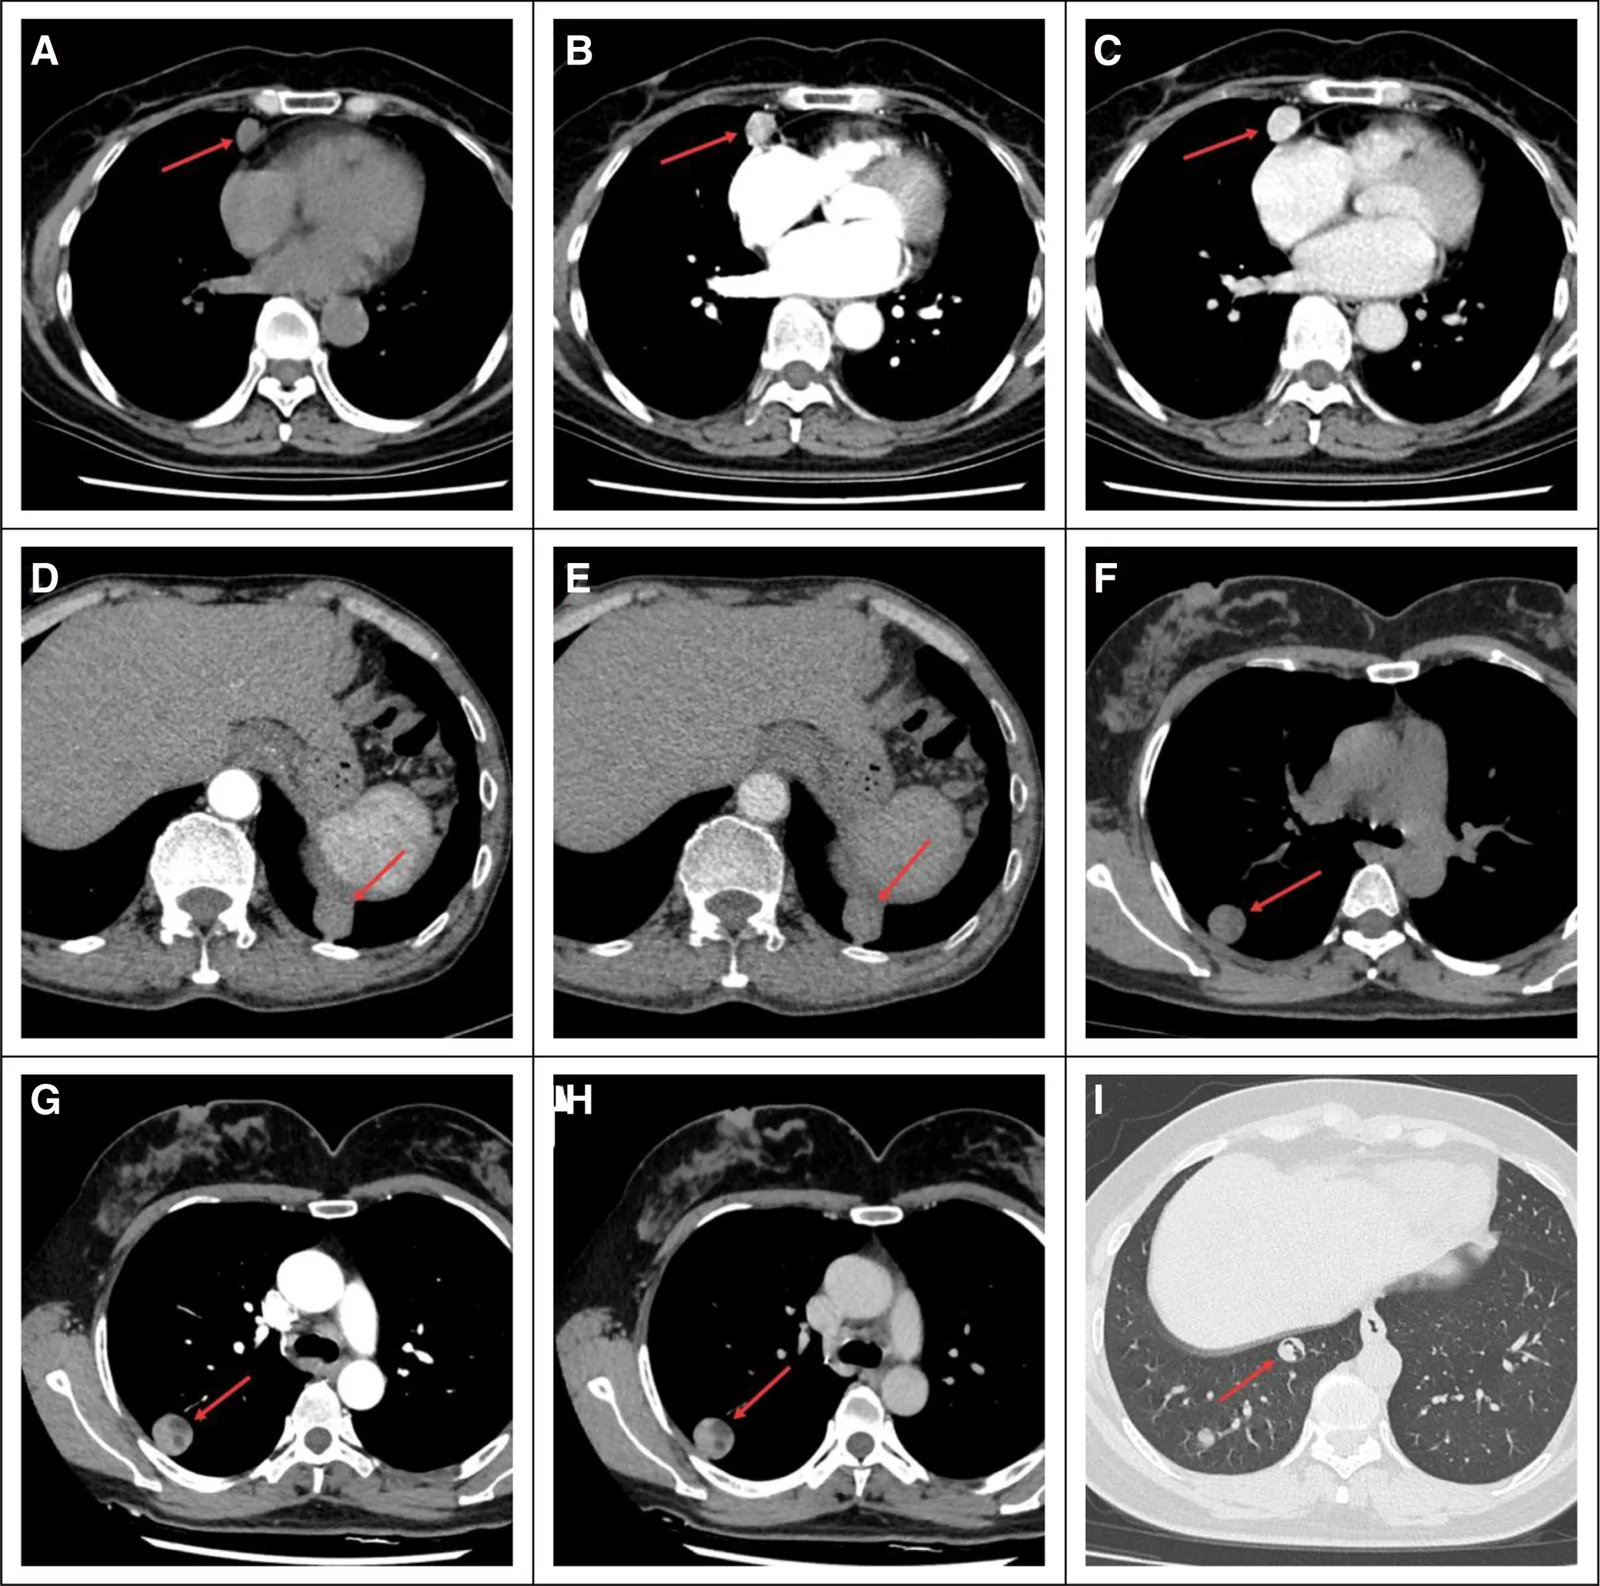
Chest CT images of pulmonary adenofibroma. Case 1 (A,B,C) A 60-year-old female. Images demonstrate chest CT mediastinal window, contrast-enhanced arterial phase, and venous phase, respectively. A well-defined solid nodule approximately 18 mm in diameter is seen in the medial segment of the right middle lobe, showing prominent but heterogeneous enhancement with more pronounced enhancement during the venous phase. Case 2 (D, E) A 66-year-old male. Images show contrast-enhanced chest CT mediastinal window during arterial and venous phases. A solid nodular shadow measuring about 26 × 20 mm is observed in the posterior basal segment of the left lower lobe. The margins are relatively smooth but poorly demarcated from the left diaphragm. Case 3 (F–H) A 44-year-old female. Images display chest CT mediastinal window, contrast-enhanced arterial phase, and venous phase, respectively. A heterogeneous round-like lesion approximately 22 mm in diameter is noted in the posterior segment of the right upper lobe. Patchy hypodense areas with no significant enhancement are visible on contrast-enhanced scans. (I) A round lesion in the apical segment of the right lower lobe, measuring about 14 mm in diameter, demonstrates internal gas-density shadows.
